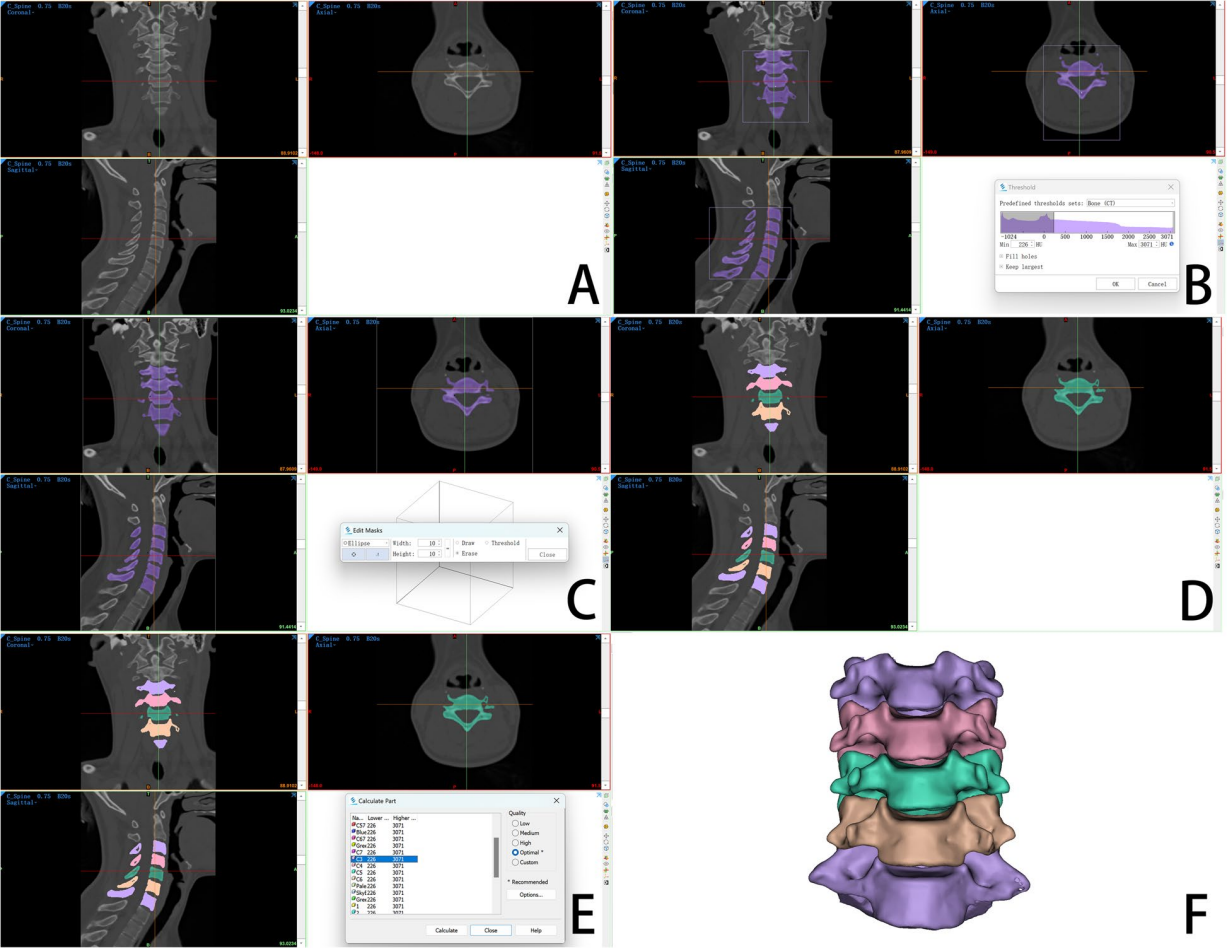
3D model reconstruction of cervical spine: import cervical spine CT image data (A), bone threshold selection (B), mask editing (C), vertebral body segmentation (D), model 3D reconstruction calculation (E), 3D models of cervical spine (F)
